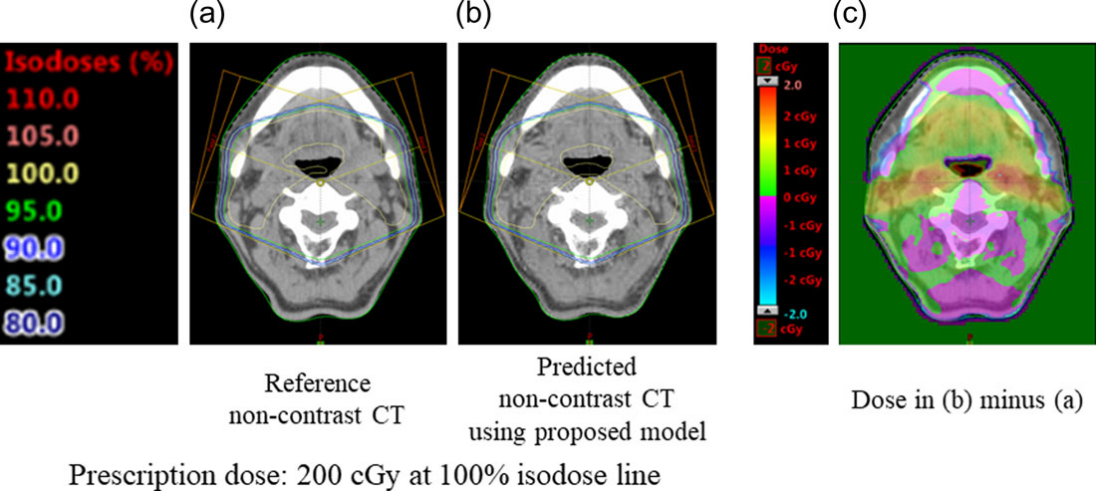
Comparison of (a) dose distributions calculated in the reference non-contrast CT and (b) dose distributions calculated in the predicted non-contrast CT using the proposed CNN model. (c) Dose difference between (b) and (a). The prescribed dose is 200 cGy. The dose in color bars ranges between −2 cGy and 2 cGy.
